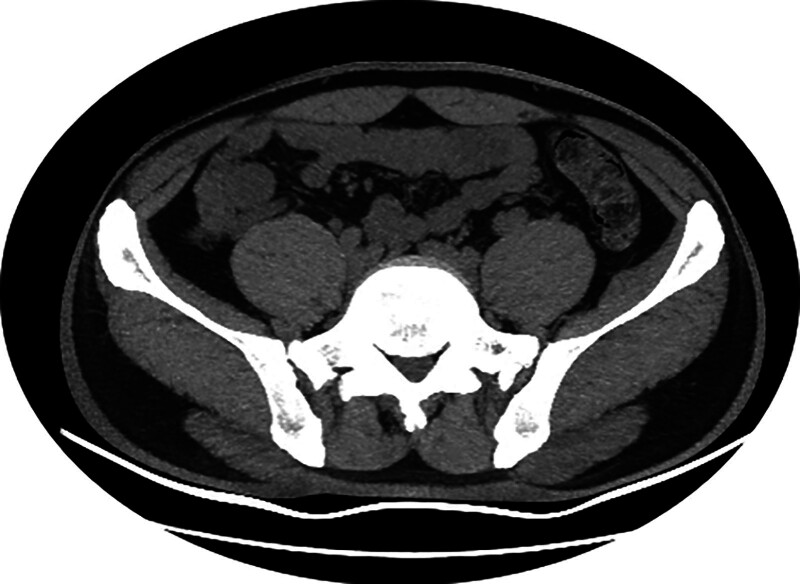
Abdominal CT scan after treatment. CT = computed tomography.
